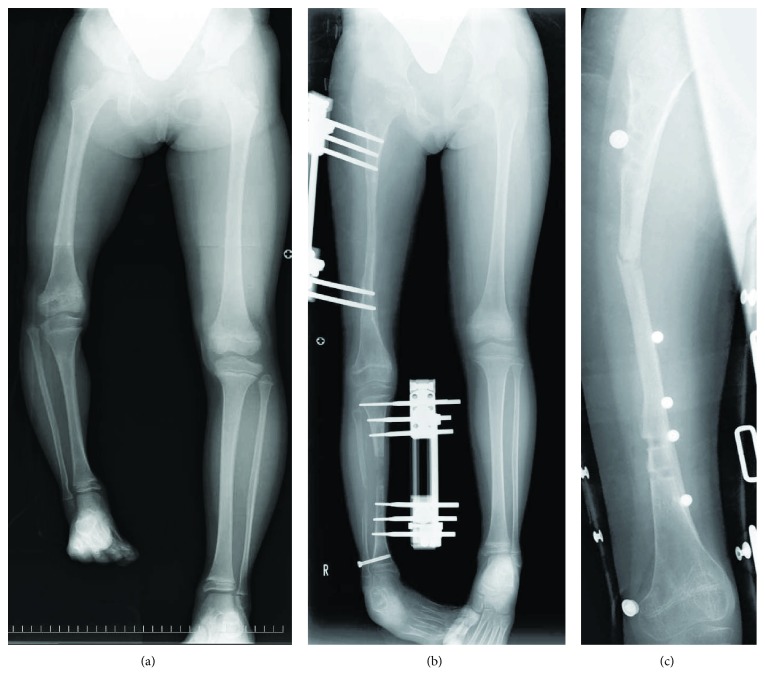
Radiographs of the lower extremities at the time of the first lengthening. (a) An anteroposterior standing radiograph of the lower extremities at seven years of age showing LLD of nearly 10 cm with the right foot placed on a block. (b) An anteroposterior supine radiograph of the lower extremities at the end of the lengthening period of the first lengthening demonstrating 83 mm and 37 mm of lengthening in the femur and tibia, respectively. (c) An anteroposterior radiograph of the right femur showing regenerate fracture at the site of the lengthened callus.
